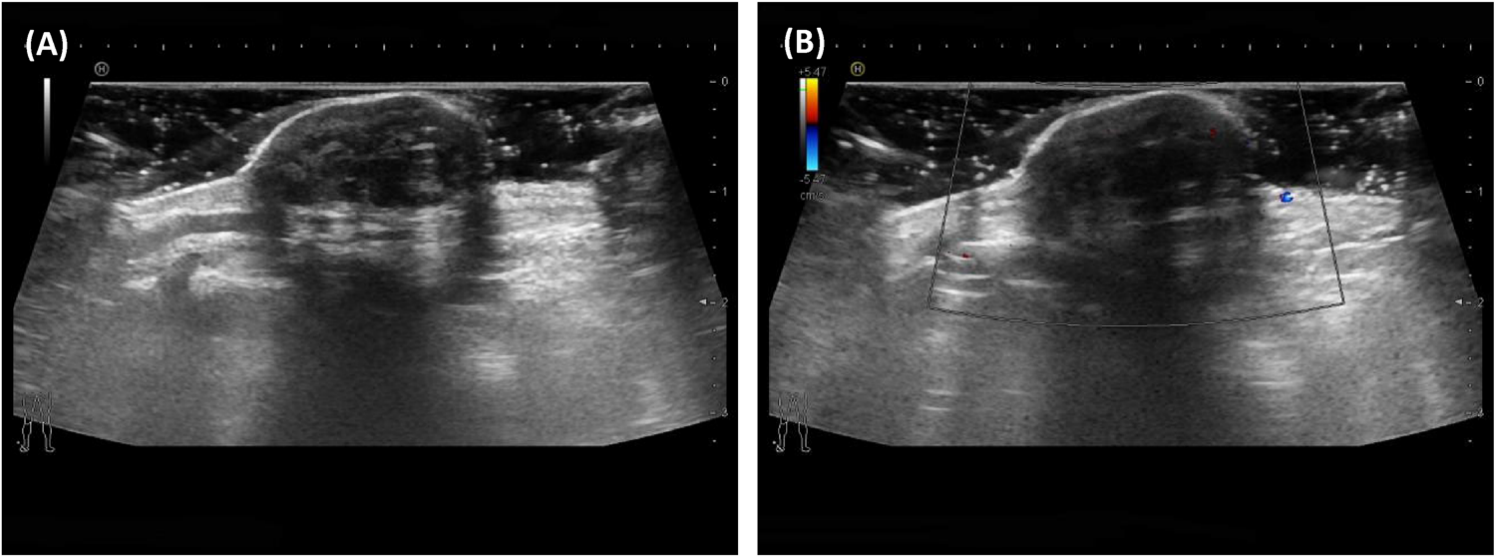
(A) High‐frequency ultrasound show a mass located in the instep skin was hypoechoic, irregular, well‐defined with internal septae. (B) Color Doppler flow imaging showed no blood flow signals within the mass.
